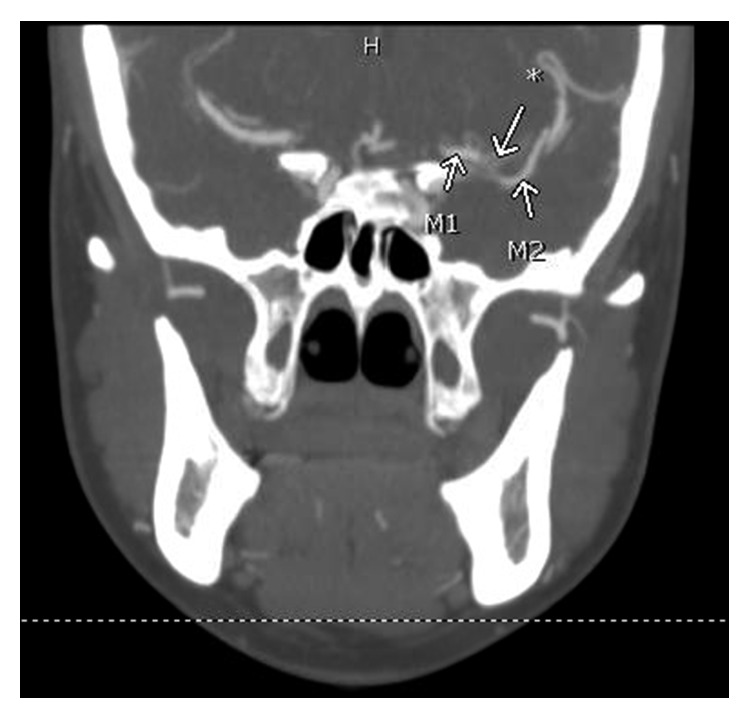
CTA neck showing a short segment of partially recanalized vessel (∗) between M1 and M2 segments of left MCA.
